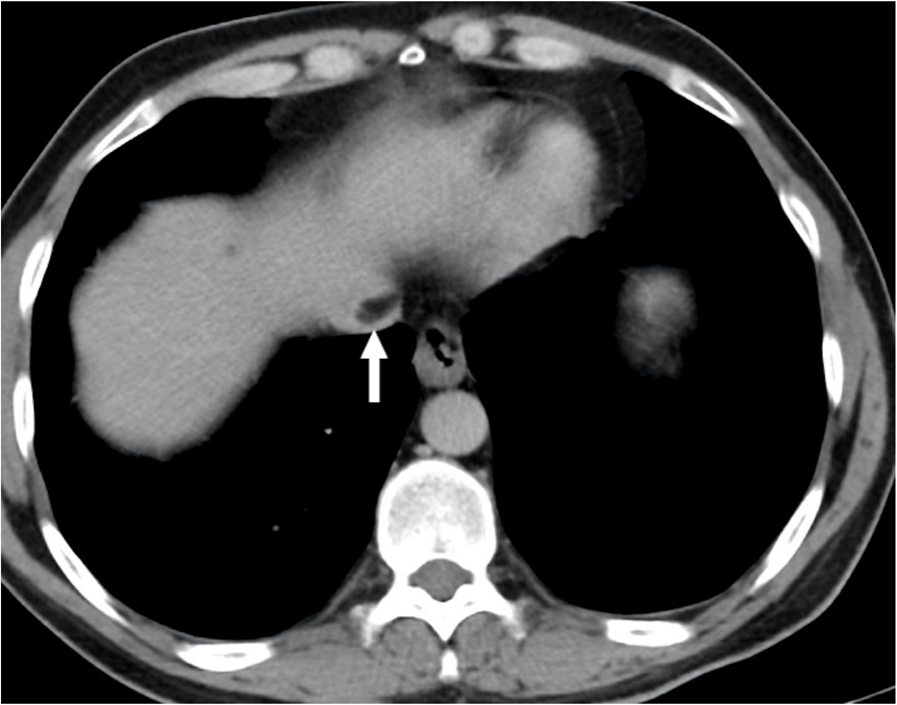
A 40-year-old male with no past medical history underwent abdominal CT examination for nonspecific abdominal pain. Axial plane postcontrast CT image demonstrates protrusion of pericaval fat into the IVC lumen (arrow) mimicking an endoluminal thrombus
